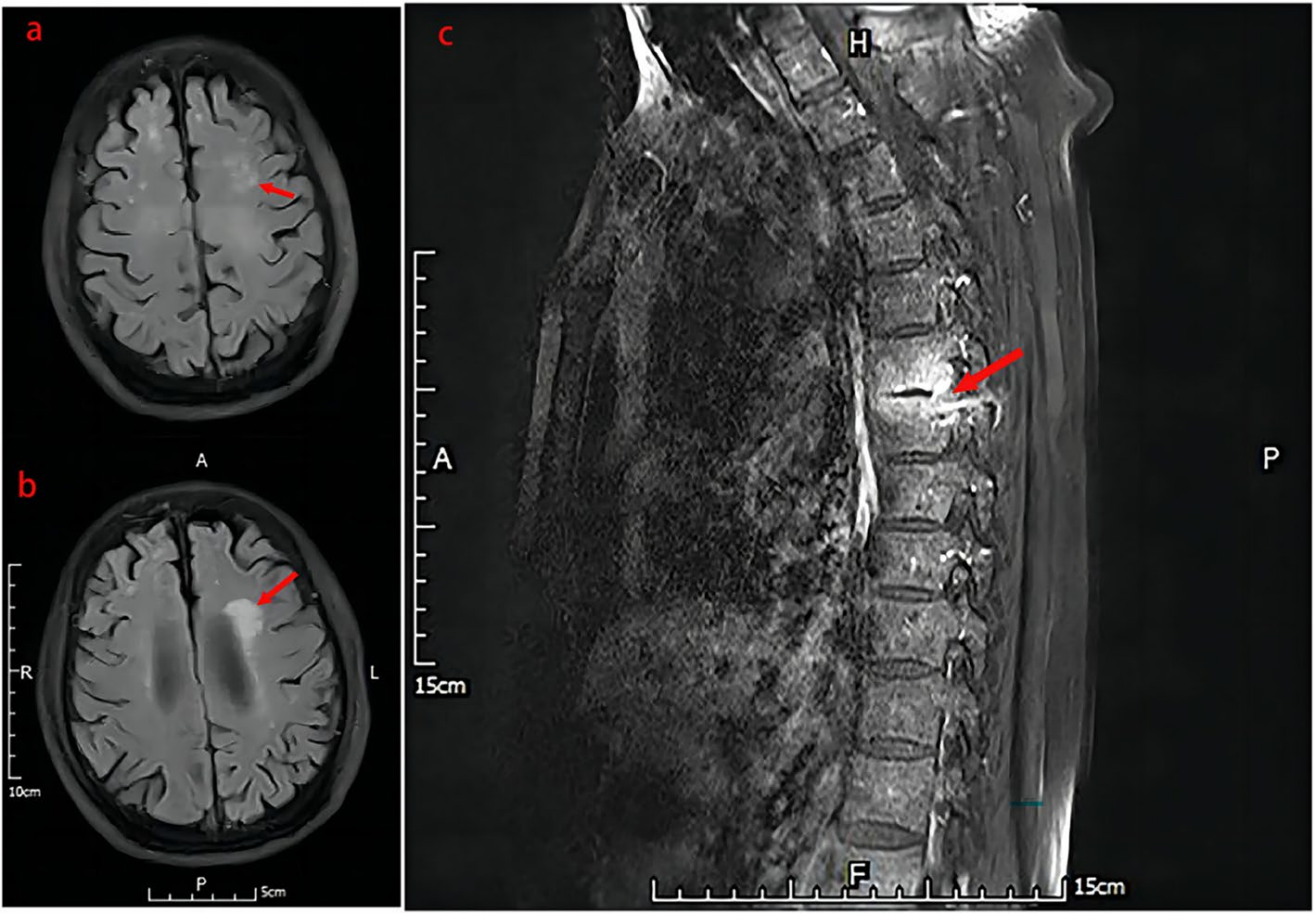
Contrast-enhanced MRI: The left temporal lobe (a) and lateral paraventricular (b) demonstrated abnormal signal and enhancement, considering infectious lesions. The thoracic vertebrae (c) demonstrated abnormal signals unevenly enhanced at T6-T7 accompanied by slight patchy enhancement of the swelling soft tissues in the vicinity
